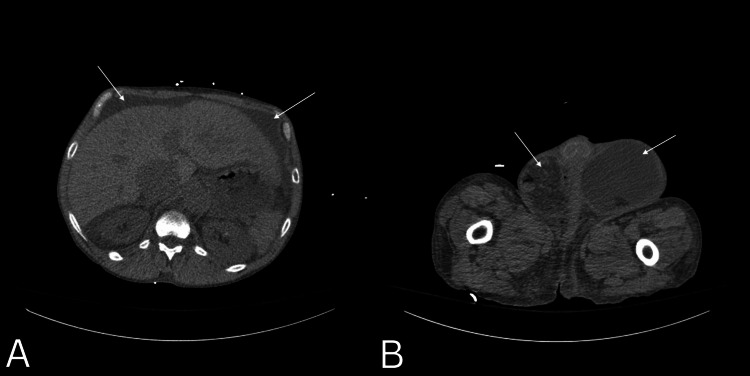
Preoperative axial computed tomography (CT) scan of a 56-year-old male patient, with ascites from heart failure. White arrows demonstrate ascites (A) and bilateral inguinal hernias (B).
